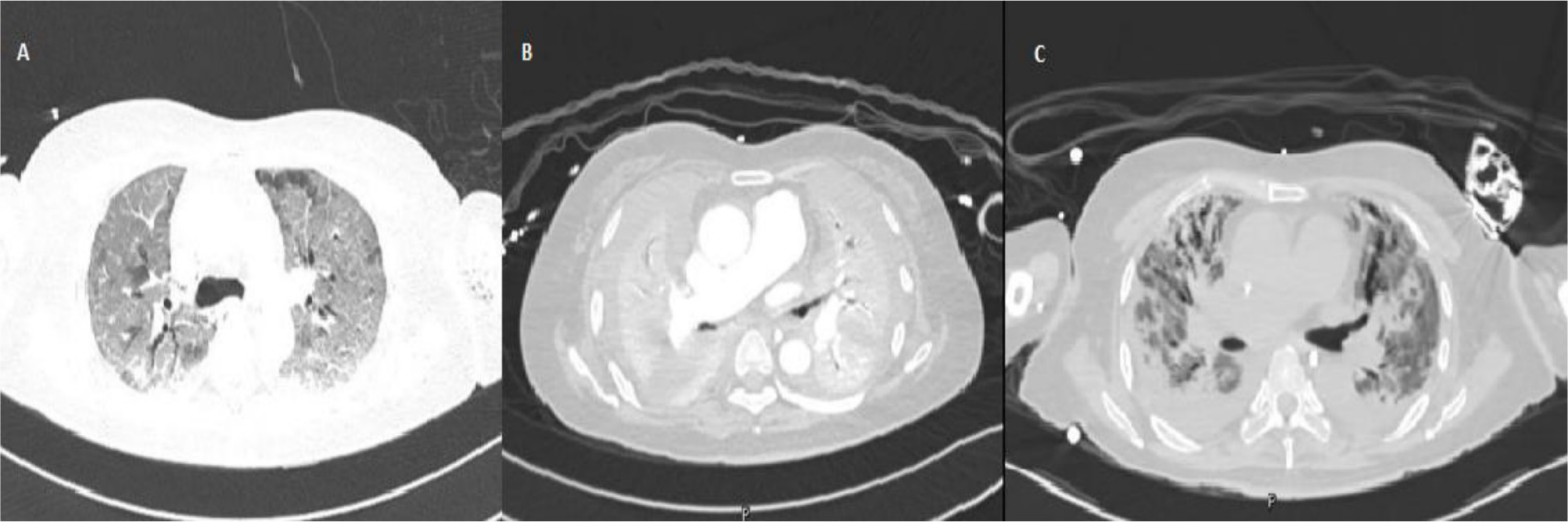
CT chest showing various phases of lung abnormalities, including bilateral ground-glass opacities (A), bilateral dense consolidations (B), and bilateral traction bronchiectasis with interstitial infiltrates (C).
